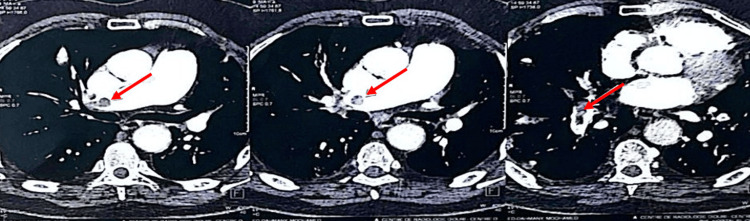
Computed tomography pulmonary angiography (axial views), with red arrows indicating bilateral proximal pulmonary artery filling defects consistent with pulmonary embolism, extending to segmental branches
